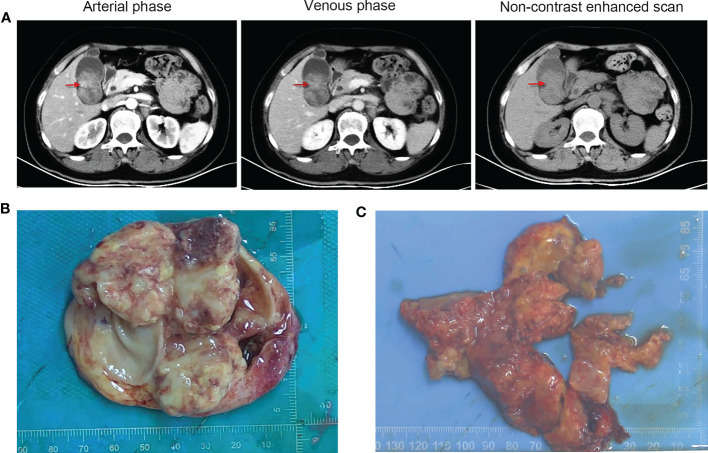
Radiographic and macroscopic images of the tumor. (A) Computed tomography images of the patient (red arrows refer to the space-occupying lesion). (B) The resected gallbladder was approximately 5.0 cm in diameter. (C) The irregular mass in the gallbladder was approximately 4.5 cm × 3.0 cm × 3.0 cm in size.
